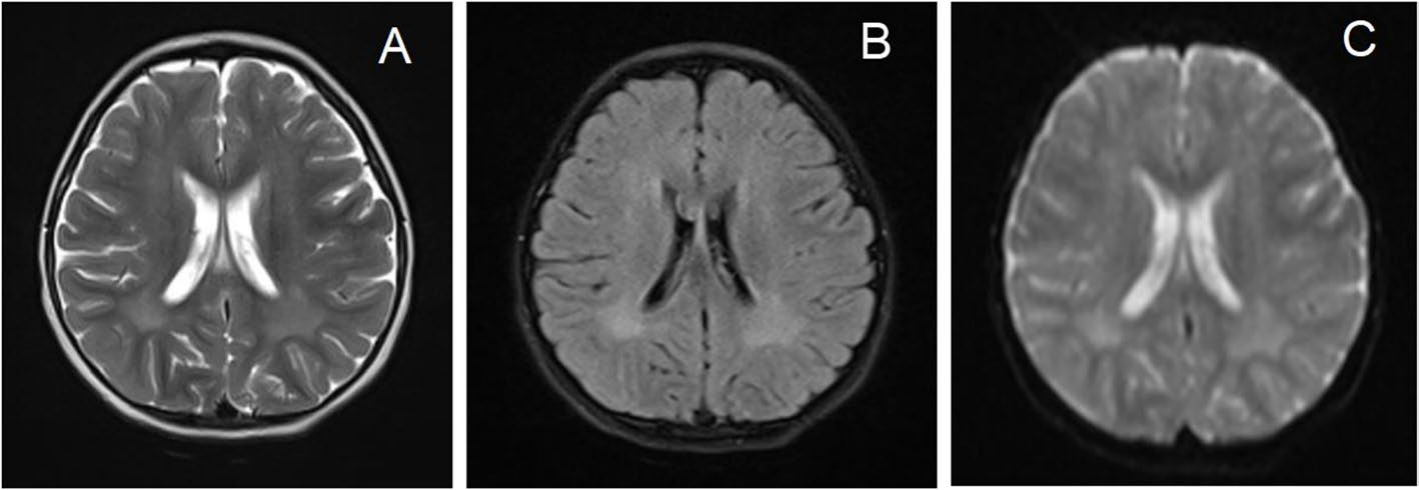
Cranial MRI results of children at the age of 3 years and 2 month. A. T2WI, B. Flair, C. DWI and T2WI images showed patchy high signal in bilateral lateral ventricles and bilateral frontal lobes. Flair was also high signal. No obvious diffusion limitation was observed on DWI
